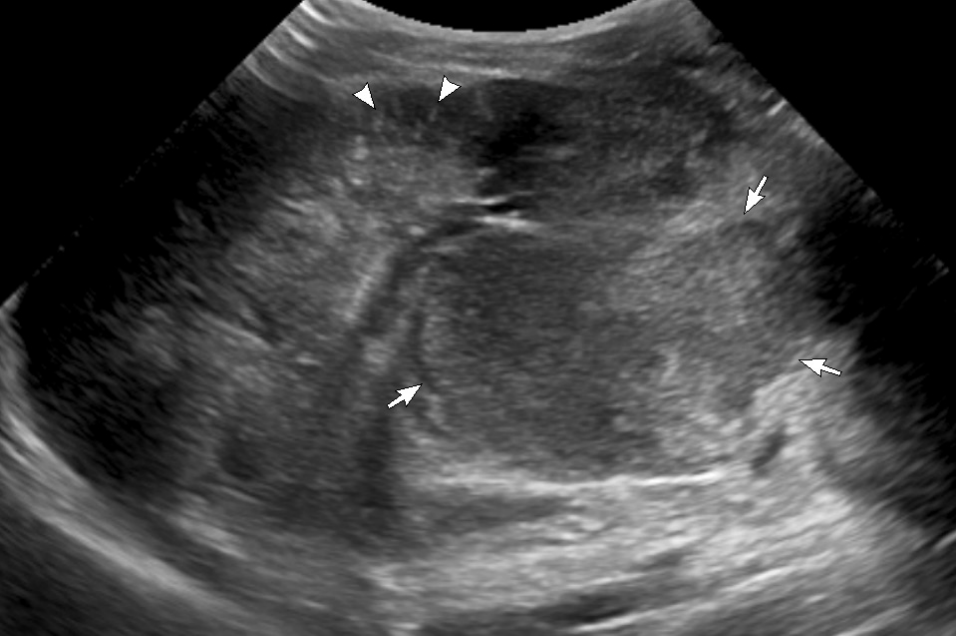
A selected ultrasound image of the left kidney showing the sizeable exophytic mass arising from the lower pole (arrows) and multiple tiny cortical AMLs (arrowheads). AML, angiomyolipoma.
